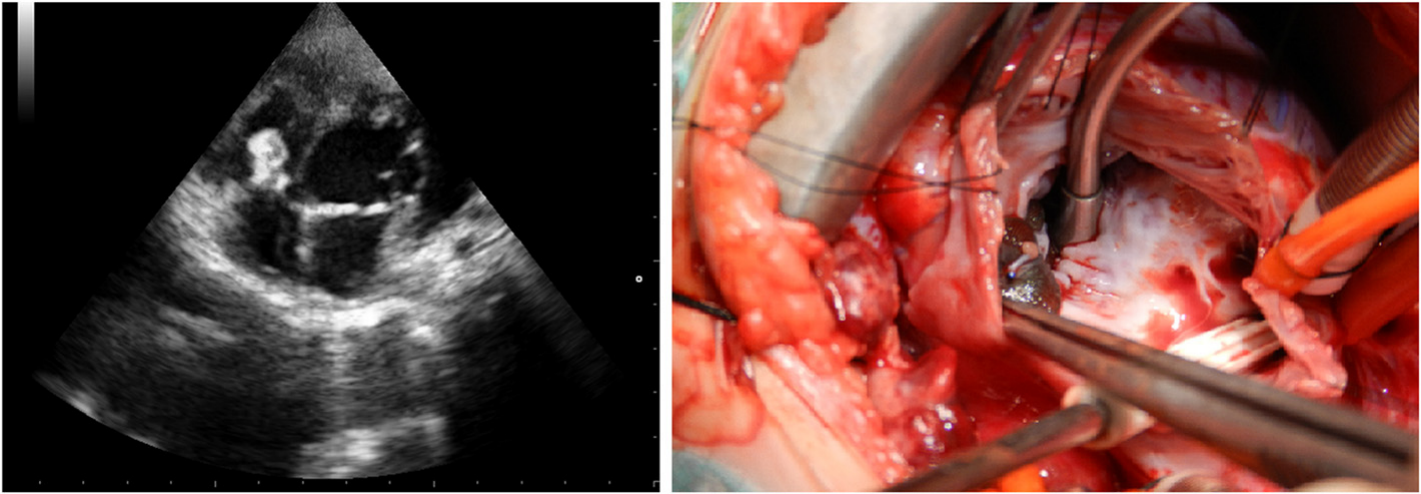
Echocardiographic and surgical views of embolised Amplatzer® ductal occluder. Device was tangled with tricuspid valve anterior leaflet chordas. Please note the ruptured chorda on the device which occurred during percutaneous retrieval attempts.
